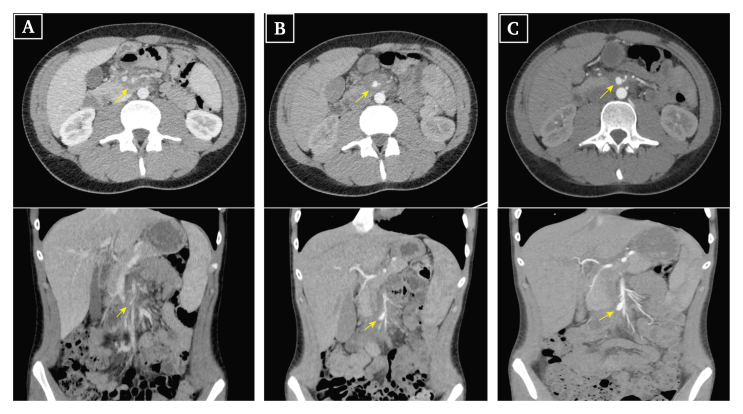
Computed tomography angiography of the superior mesenteric artery in axial and coronal sections showing the evolution of the pseudoaneurysm (yellow arrow) on (A) day 0, (B) day 2, and (C) day 7 of the hospital stay.
